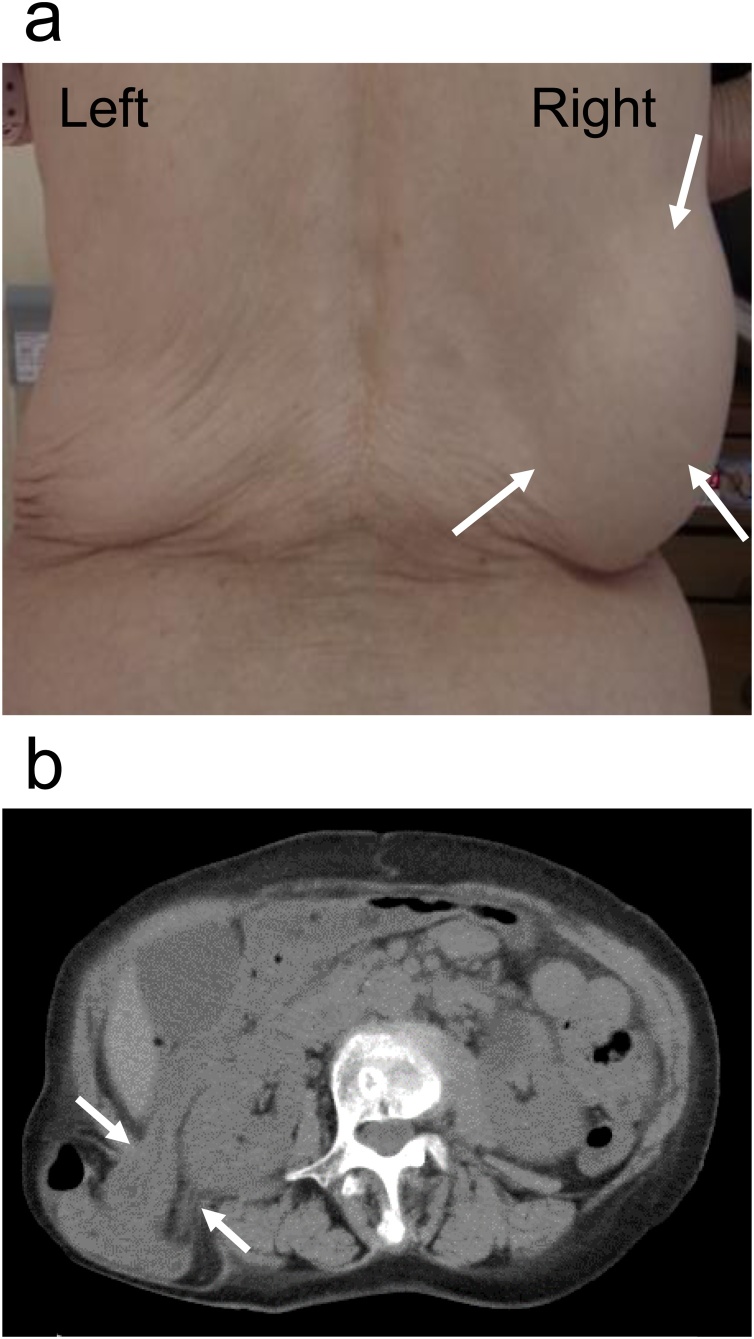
Physical and radiologic images of the right superior lumber hernia. (a) Physical findings in the standing position. (b) Computed tomography imaging showing right superior lumbar hernia. (a) A reducible bulge in the right upper back can be seen (arrows). (b) Herniation in the right superior lumbar space. The hernia is assumed to contain the ascending colon (arrowheads).
